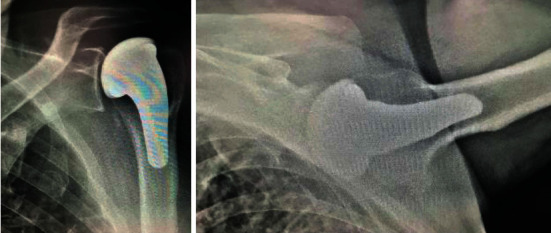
Plain radiographs of the left shoulder with good implantation of the humeral steam and no evidence of glenoid erosion.
